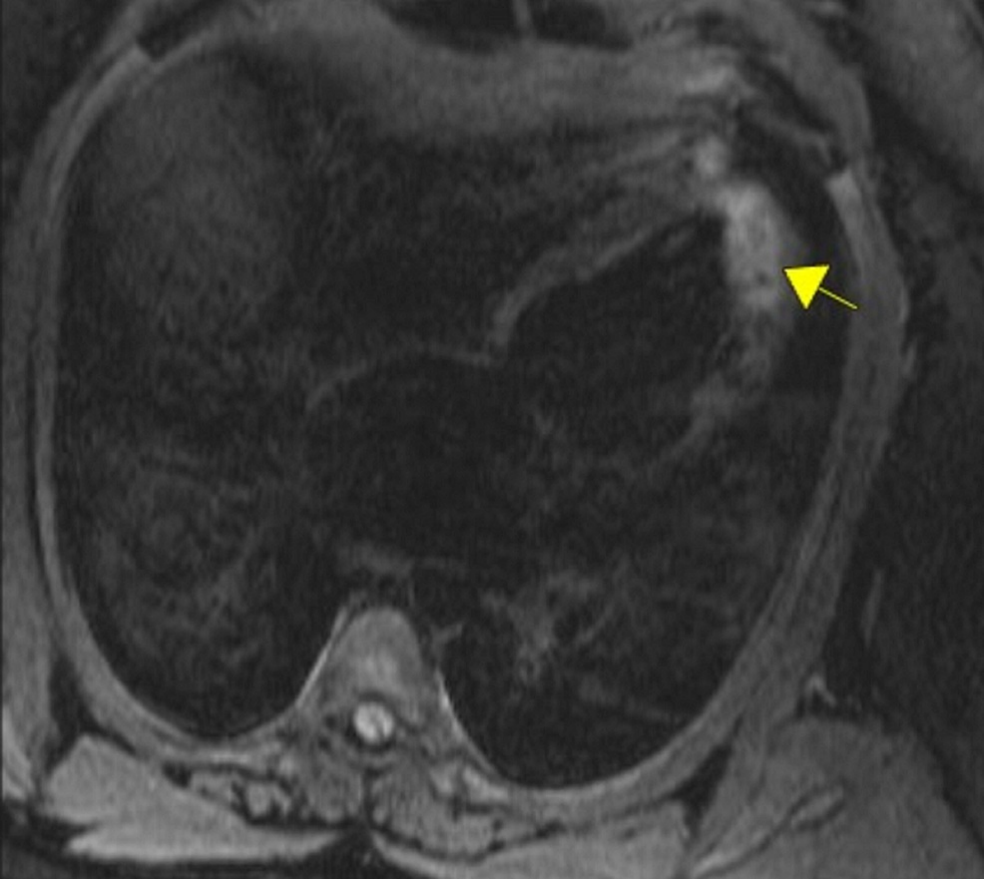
Cardiac MRI showing myocardial enhancement of left ventricle on T2 images concerning for myocardial inflammation. MRI: magnetic resonance imaging
